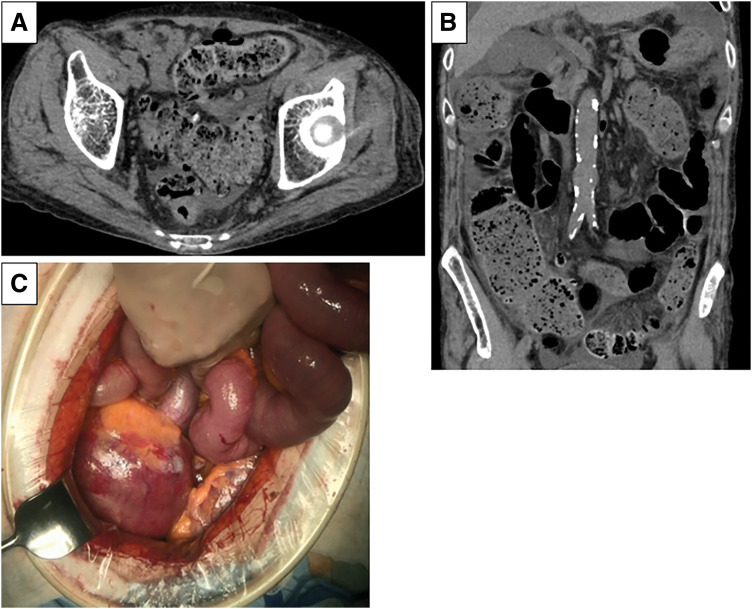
Intraoperative findings in Case 2. (A, B) CT demonstrated fecal impaction extending from the ileocecal region to the sigmoid colon, with pneumatosis intestinalis in the sigmoid colon. (C) Intraoperative findings revealed marked dilatation of the cecum with poor coloration, as well as discoloration around the splenic flexure.
